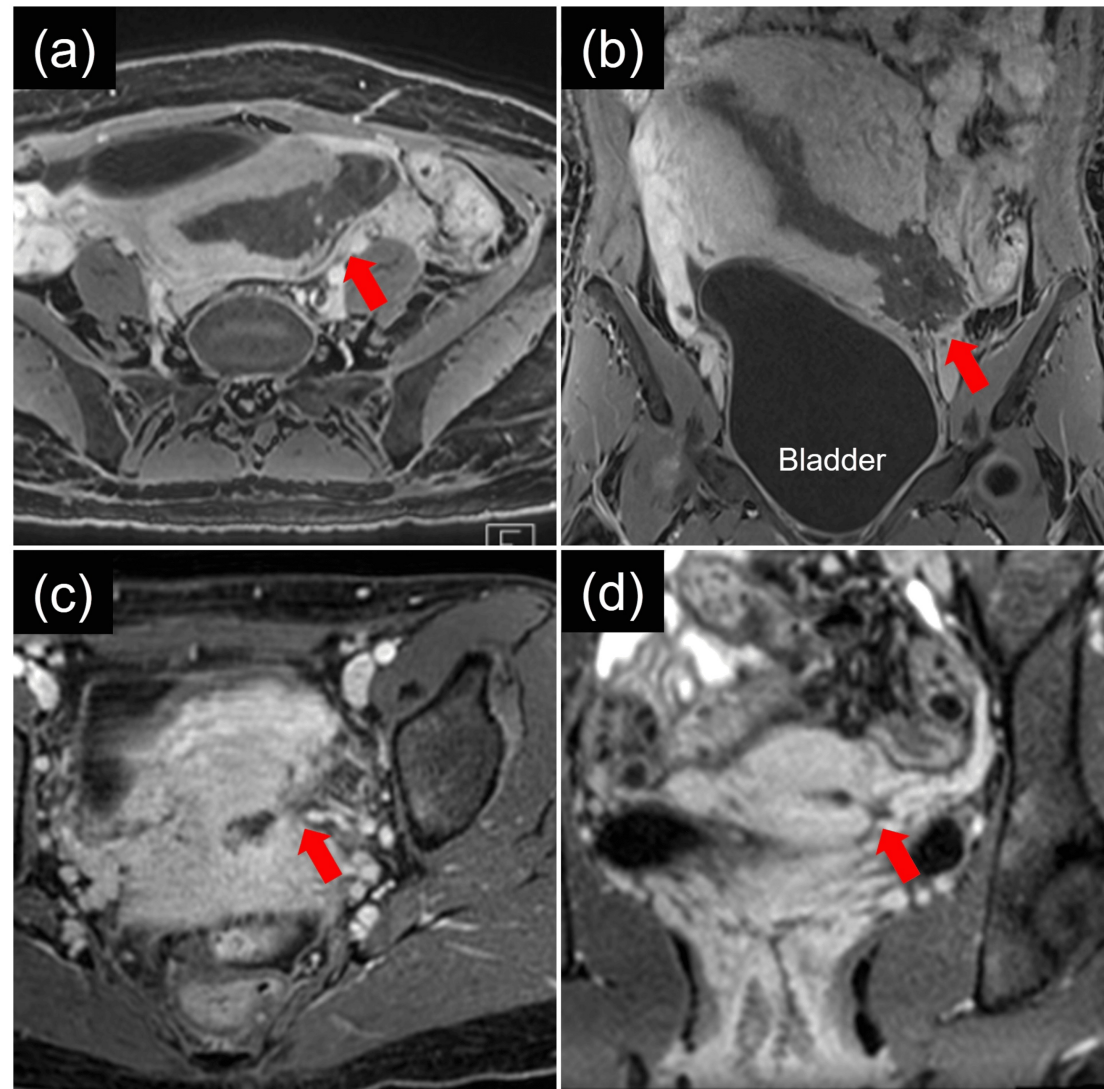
Magnetic resonance imaging of the previous uterine rupture. (a, b) Axial (a) and coronal (b) T1-weighted images immediately after the uterine rupture. A disruption in the myometrium is observed on the left side of the lower uterus. The broad ligament is preserved, leading to a diagnosis of incomplete uterine rupture. The red arrows indicate the uterine rupture site. These images have been revised from those published in our previous report. (c, d) Axial (c) and coronal (d) T1-weighted images at one year after the uterine rupture. Significant thinning of the myometrium is observed on the left side of the lower uterus, but the continuity is maintained. The red arrows indicate the uterine rupture scar.
